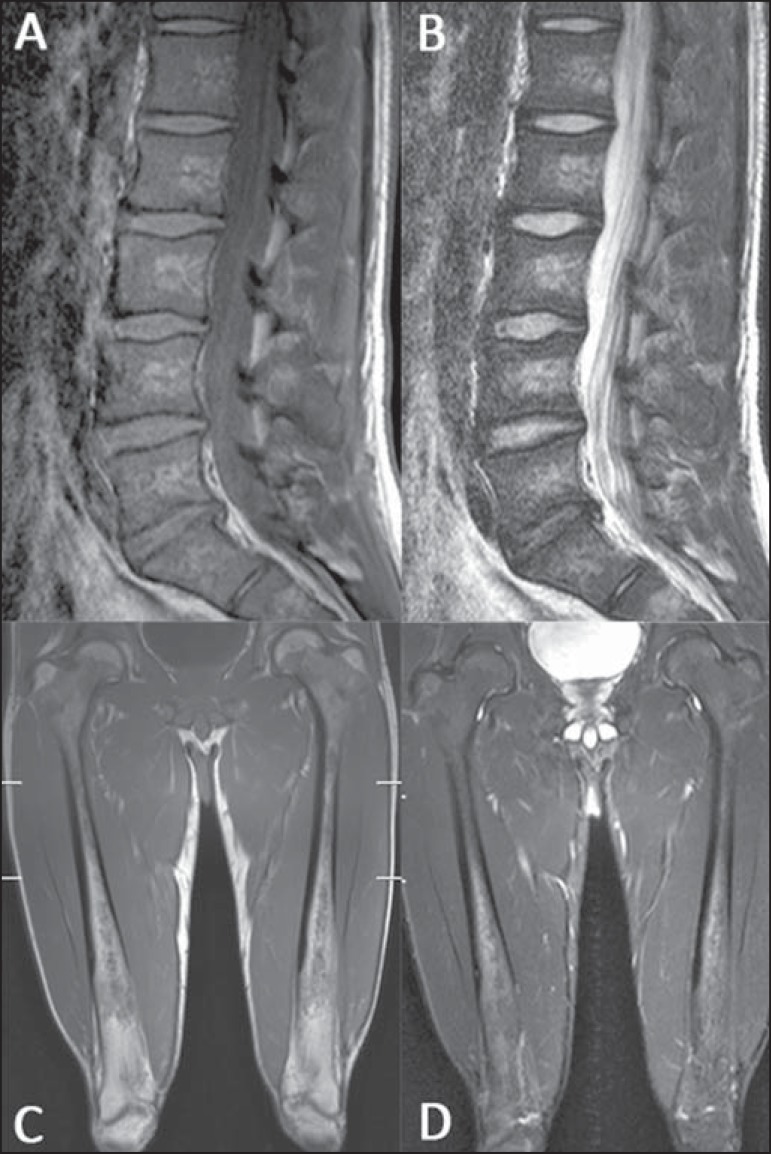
MRI of a male, 28-year-old patient presenting with Gaucher’s disease. T1-weighted
